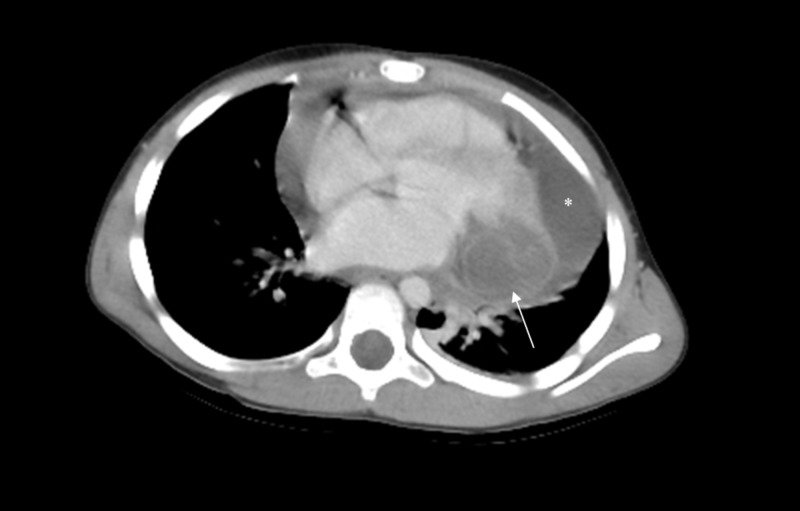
CT of the Heart CT scan of the heart demonstrating pericardial effusion (asterisk) and the presence of cystic lesion involving the left ventricular wall (arrow)
